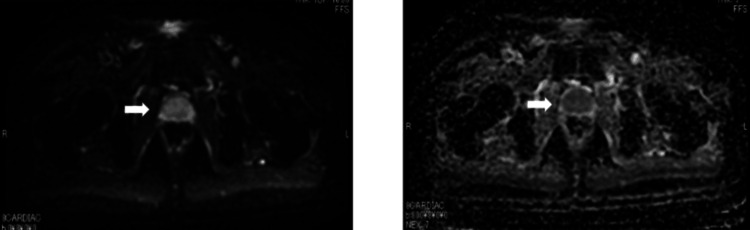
MRI imaging of the prostate at 14 months after starting triplet therapy Left: diffusion-weighted imaging (DWI); Right: apparent diffusion coefficient (ADC) map
